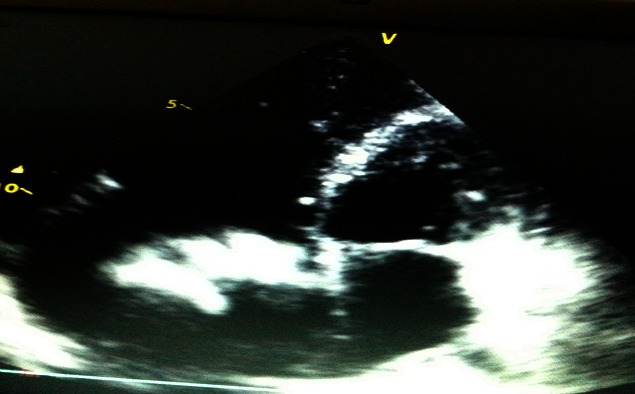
Transthoracic echocardiography showing a right atrial polyploidy mass, right atrial and ventricular dilatation
